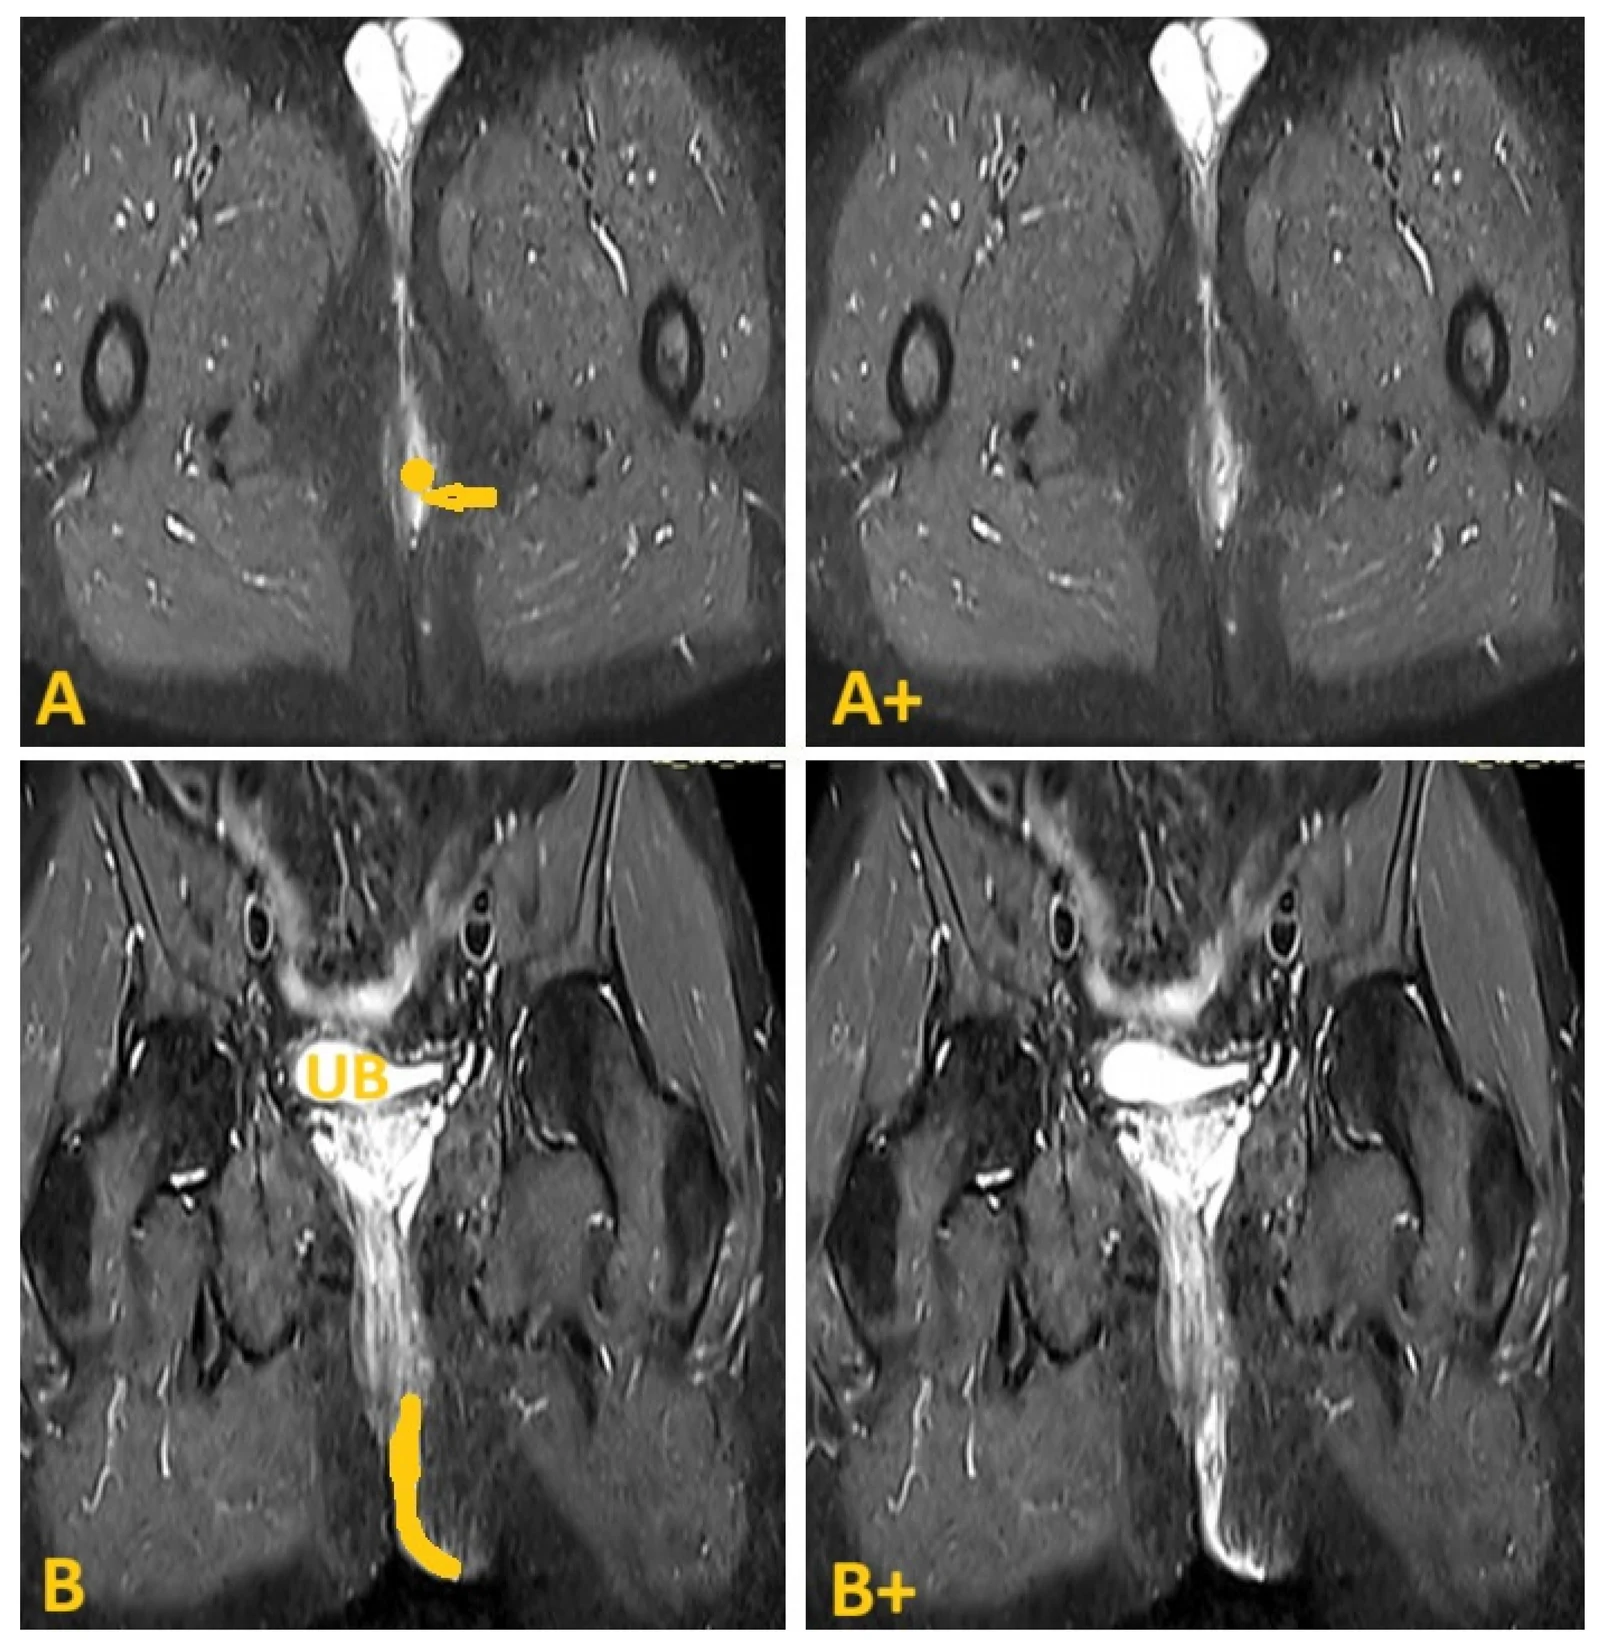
Selected magnetic resonance imaging images. (A) Axial STIR T2-weighted image showing a perianal fistula arising from the left posterior anal sphincter region at the 5 o’clock position (arrow) around the anal canal (dot) in the lithotomy position, with the internal opening located 20 mm from the anal verge. (A+) Corresponding unannotated image. (B) Coronal STIR T2-weighted image showing an intersphincteric fistulous tract measuring 53 mm in length and 8 mm in width, extending inferiorly in the left posterior quadrant to form an external opening. No branching or abscess formation is identified. (B+) Corresponding unannotated image. UB: Urinary bladder.
